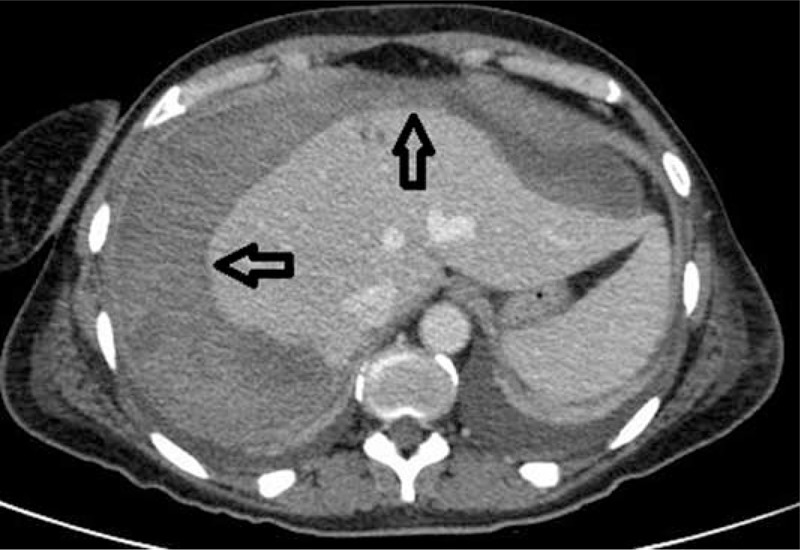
CT scan of upper abdomen showing a reduction of subcapsular hepatic hematoma (hypodense area indicated by the arrows), mainly on the surface of the right lobe of liver. There are no sources of active bleeding. CT = computed tomography.
